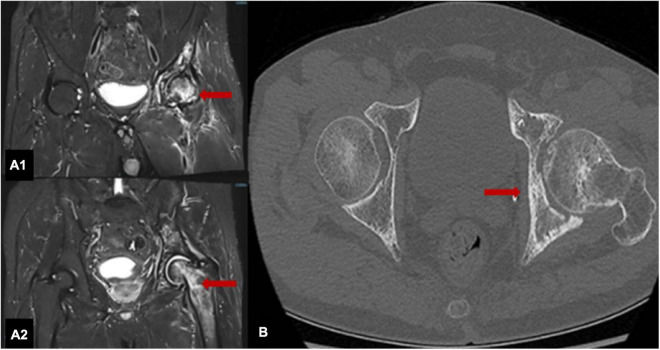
MRI scans of both hips show that the shapes of the left femoral head and upper femur are normal, with patchy T1 and T2 signals, uneven signals and patchy low-signal areas; the right hip joint adjacent bone shows uniform signals (A1,A2). The left hip joint is narrow, and the articular surface is not smooth; the left femoral head is slightly flattened with a patchy high-density shadow with an unclear boundary (B).
